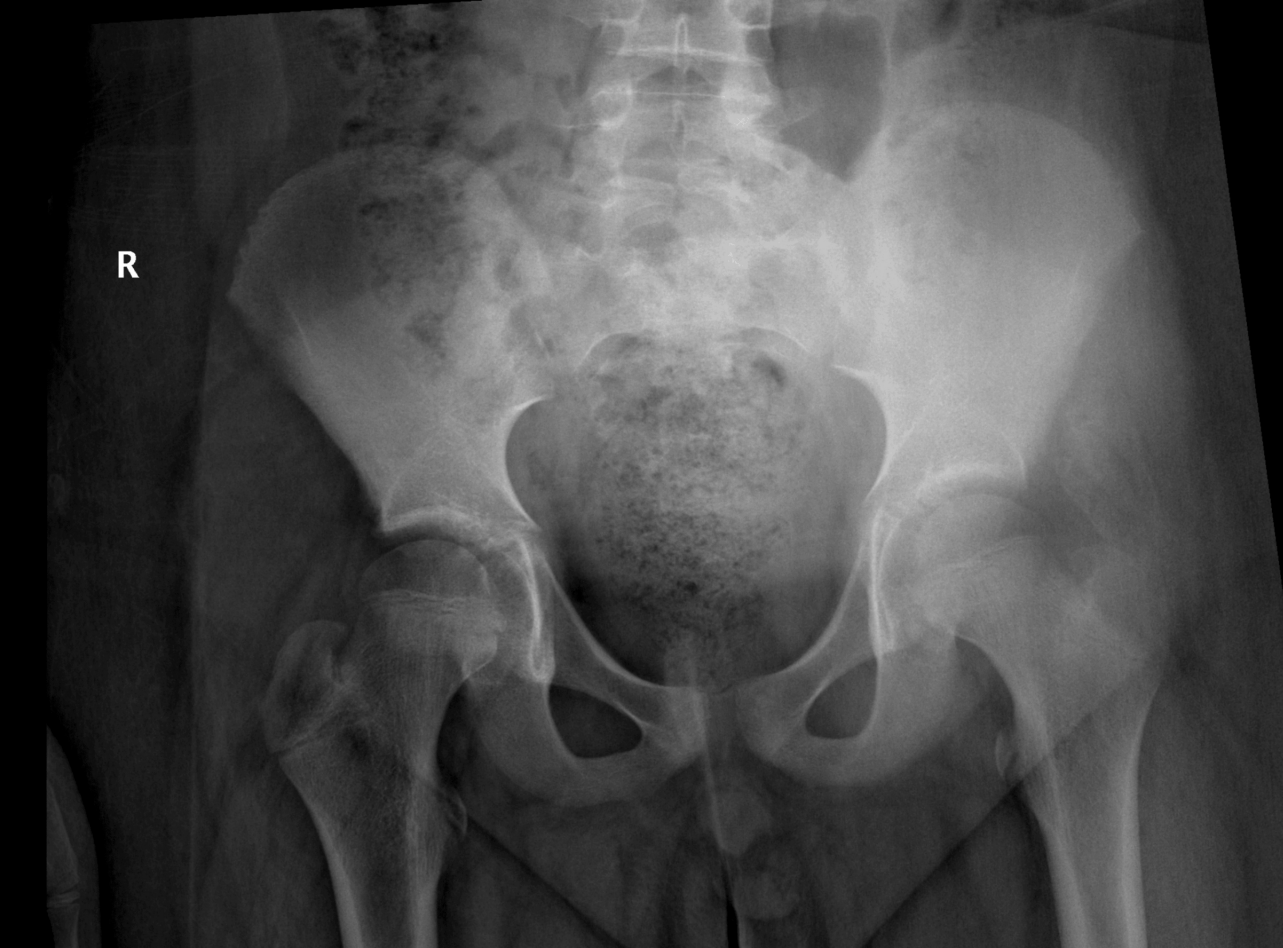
AP pelvis X-ray three months following presentation
